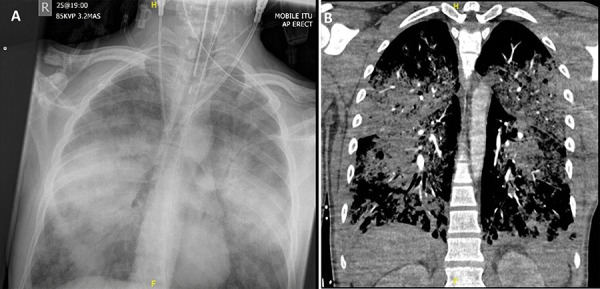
A: Repeat chest radiograph and B: CT pulmonary angiogram performed after intubation.
